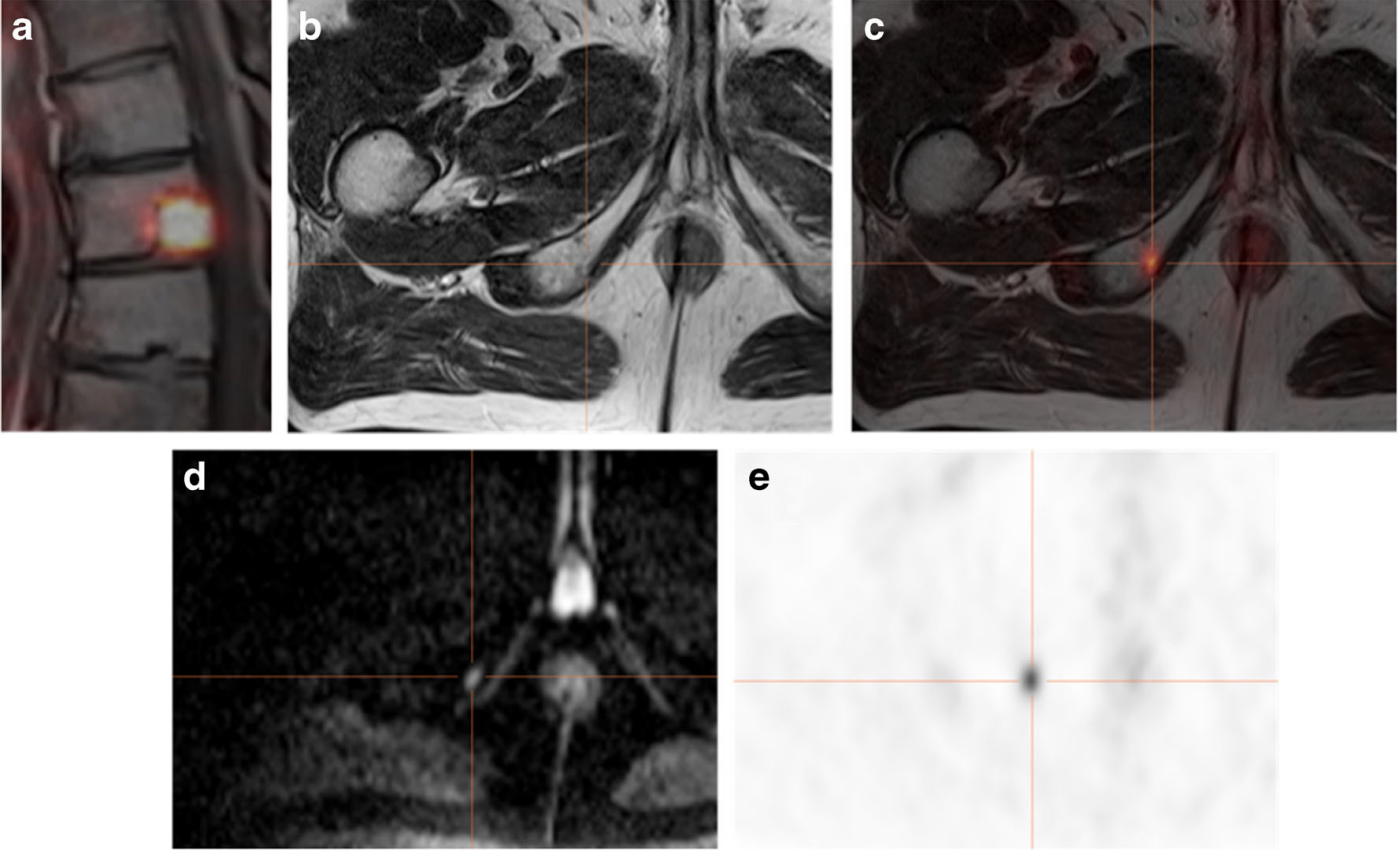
High sensitivity of [^68^Ga]Ga-PSMA^HBED-CC^ conjugate 11 (PSMA)-PET and diagnostic benefit by simultaneous PET/MRI in a 68 y/o patient after radical prostatectomy and radiation therapy of the prostate bed. PSA relapse of 1.7 ng/ml at the time point of PSMA-PET/MRI. Radiation therapy was planned in the thoracic vertebra (a; also prospectively positive on MRI) but was then extended to the ischiadic arc. The additional lesion is not seen on T2w sequences (b), but the focal PSMA uptake (c+e) was also confirmed by a focal diffusion restriction on diffusion-weighted b600 sequences (d). PSA turned to <0.2 ng/ml after RT
